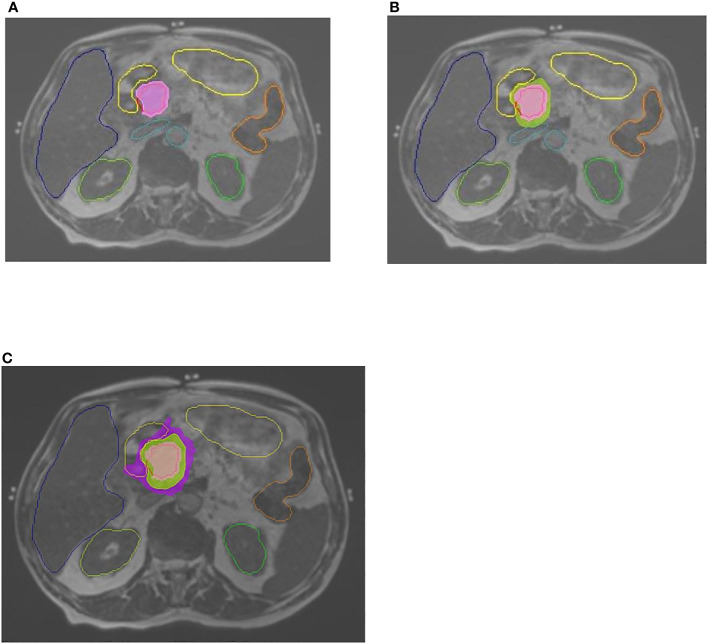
Contouring and doses. Abdominal MRI, 0.35T, with contoured OARs: stomach-duodenum, liver, kidneys, large bowel, great vessels. (A) GTV contoured in red. Pink color wash–100% dose (of 50 Gy prescribed dose). (B) Green color wash—95% dose. (C) Purple color wash—50% dose.
